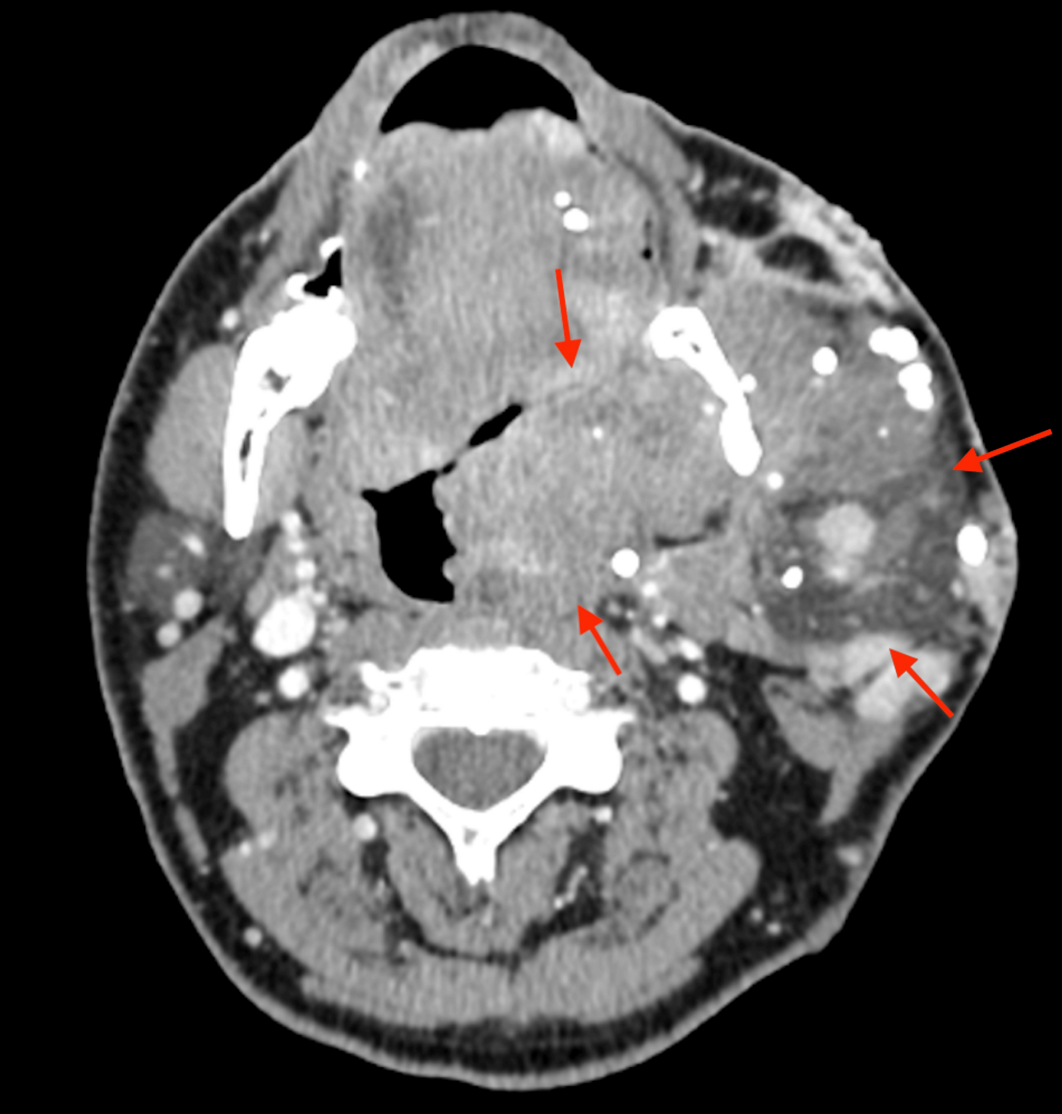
Axial view of a CT scan of a neck at the C2 level of the oropharynx depicting a large left-sided haemangioma extending deep in to the airway indicated by the red arrows CT: Computed tomography
